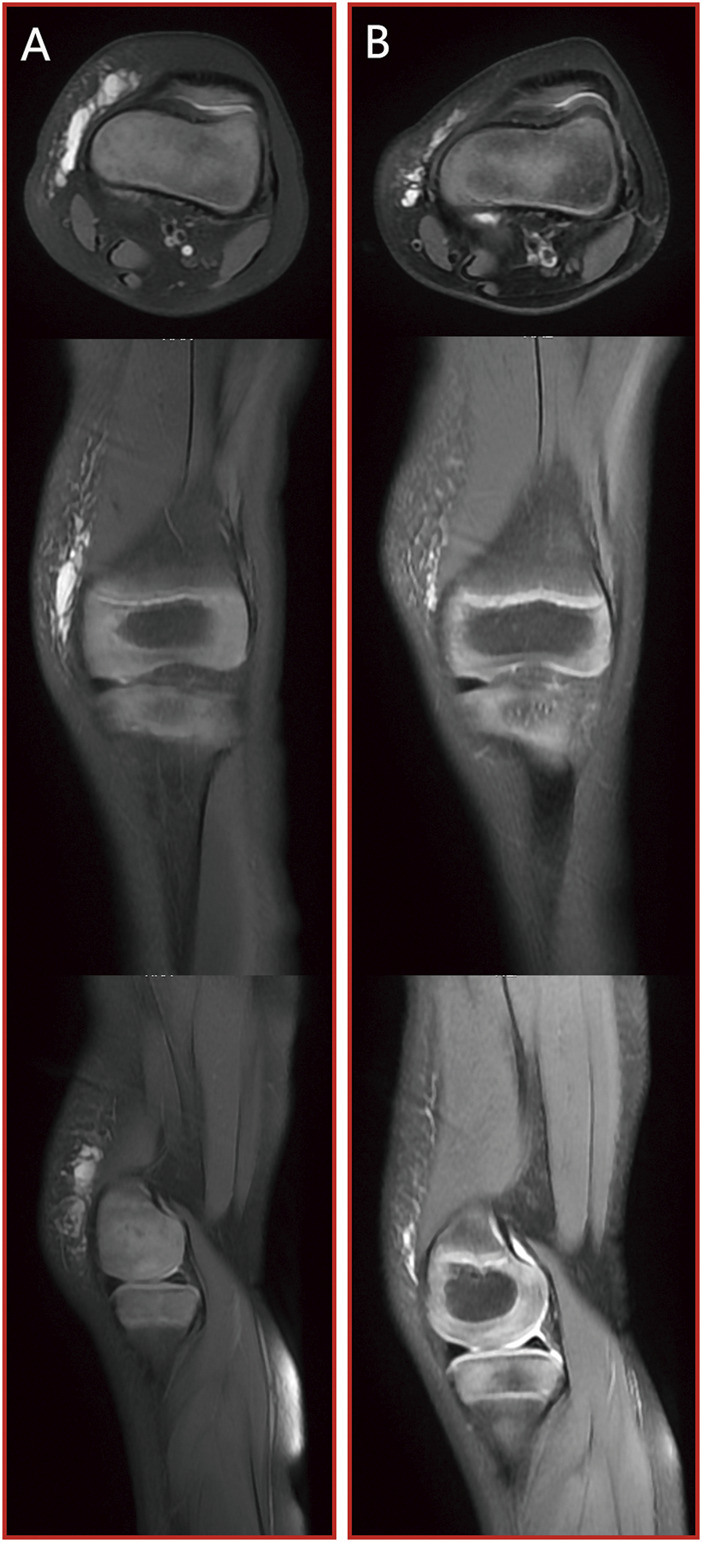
Case 2: patient with microcystic LMs of the left distal medial thigh which achieved a notable reduction in the size of the intraconal cystic component after two sessions of combination therapy. (A) pre-treatment MRI stud; (B) post-treatment MRI study. From top to bottom: axial T2 fat saturated image, coronal T2 fat saturated images and sagittal T2 fat saturated images.
